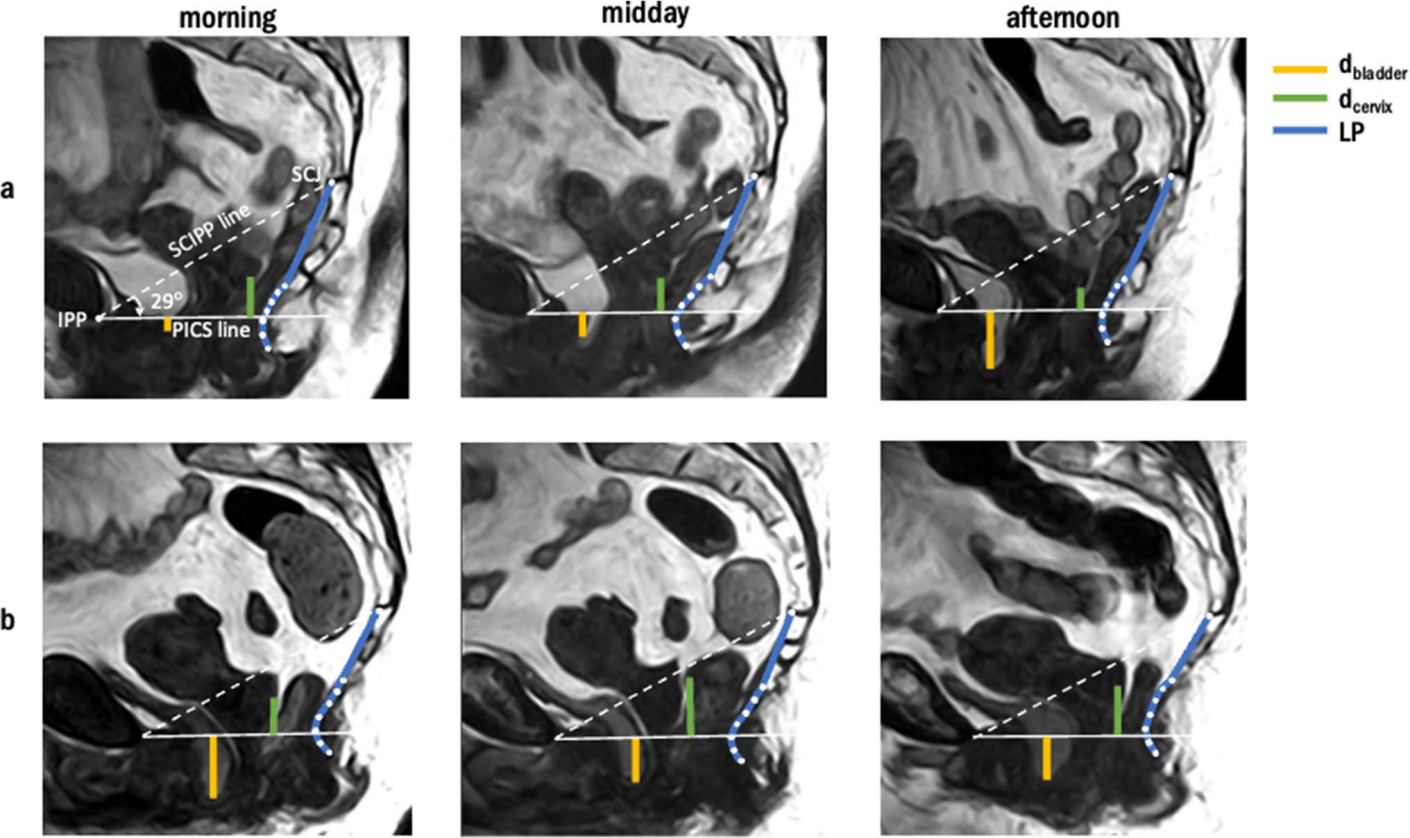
Sagittal magnetic resonance images of two pelvic organ prolapse (*POP*) patients at the three moments during the day, on which distance from lowest point of the bladder to the pelvic inclination correction system (*PICS*) line (d_bladder_), distance from the posterior point of the cervix to the PICS line (d_cervix_), and the levator plate (*LP*) were visualized. a The bladder descended from 0.6 cm below the pelvic inclination correction system (*PICS*) line in the morning to 2.8 cm below the PICS line in the afternoon. The cervix descended from 1.4 to 1.0 cm above the PICS line. b The bladder ascended from 3.2 to 2.4 cm below the PICS line, the cervix ascended from 1.6 to 2.2 cm above the PICS line. *IPP* inferior pubic point, *SCJ* sacrococcygeal joint, *SCIPP* sacrococcygeal to inferior pubic point line
